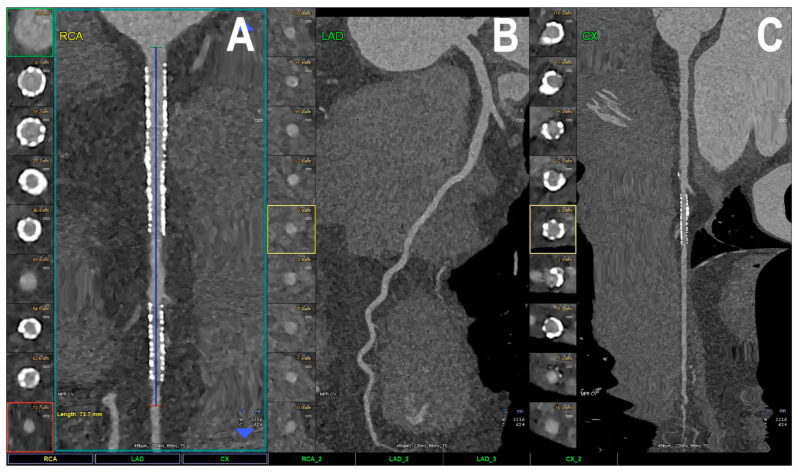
Cardiac PCCT visualization of coronary stents and stented lumen. There are two stents at the level of the proximal and middle RCA (A) and one stent on the marginal branch of the left LCx (C); the LAD (B) is normal, without any detectable atherosclerotic disease. All stents are perfectly visualized in terms of their inner struts and also in their inner lumen, which is difficult to achieve using standard cardiac CT. PCCT—photon-counting CT, LAD—left anterior descending, LCx—left circumflex, RCA—right coronary artery. Reprinted with permission under open access from Cademartiri et al. [22].
